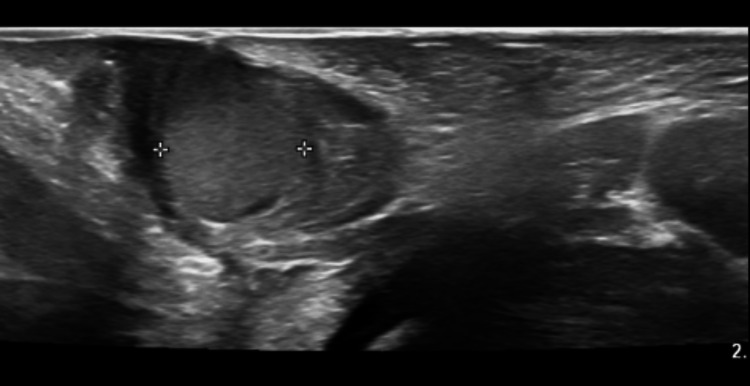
Transverse translabial ultrasound view Transverse translabial view showing the 1.1 x 1.0 x 1.0 cm mass in the vaginal canal. It is homogeneous and isoechoic. There is mild posterior acoustic enhancement. The presence of echogenicity and absence of an anechoic center made the lesion appear solid rather than cystic, which may have contributed to its misinterpretation as testicular tissue.
